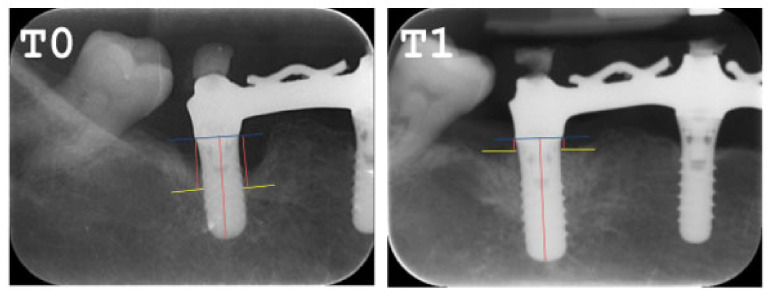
Periapical radiograph pre and post. T0: Periapical Rx pre regenerative surgery in peri-implant defects. T1: Periapical Rx pre regenerative surgery in peri-implant defects.
